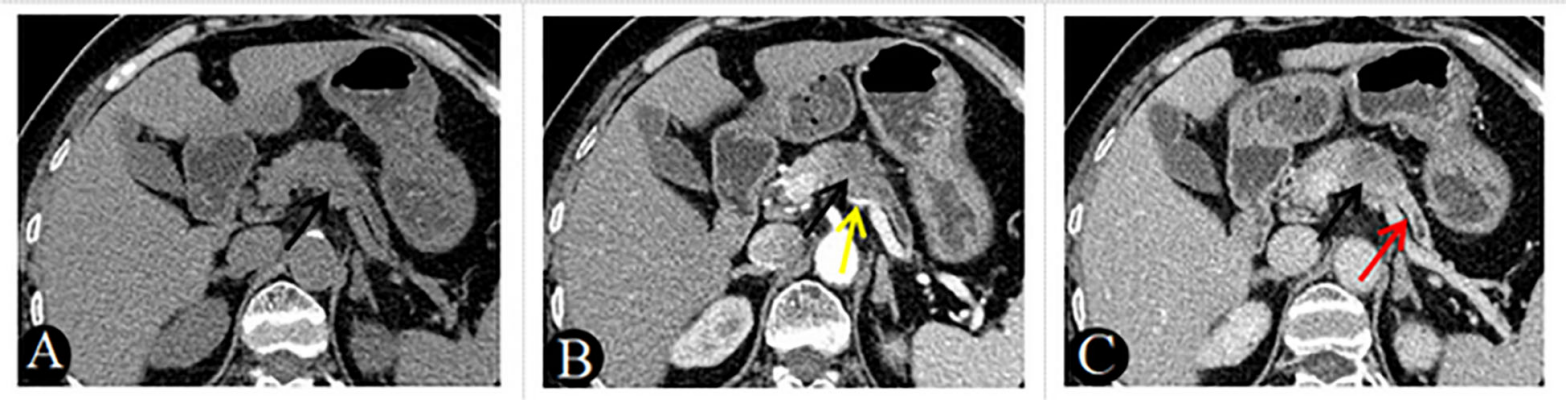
A 69-year-old woman with PDAC, absence of clinical symptoms, CA199 positive. Axial unenhanced (A), pancreatic parenchymal (B), and portal (C) phases CT images, demonstrated a low-attenuation nodules approximately 25mm in diameter in the neck of the pancreas (black arrow). The lesion showed gradual enhancement type and ill-defined margins, associated with main pancreatic duct dilatation (C, red arrow) and atrophy of the body and tail of the pancreas (A–C). Note also the involvement of the splenic artery, which is narrowed (B, yellow arrow).
